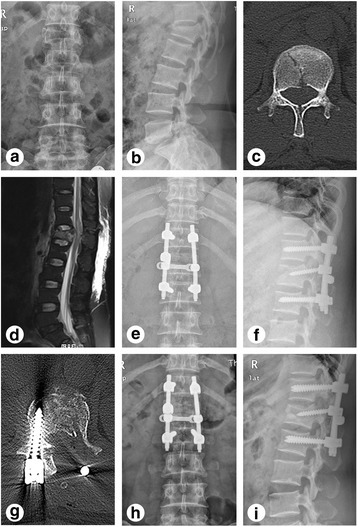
Preoperative, postoperative, and a 3-month follow-up radiographs of a patient with thoracolumbar burst fractures and greenstick lamina fractures treated with the open book laminectomy approach (case 21). a–d X-ray, CT, and MRI before operation. e–g X-ray and CT at 3 days after operation. h, i X-ray at a 3-month follow-up
